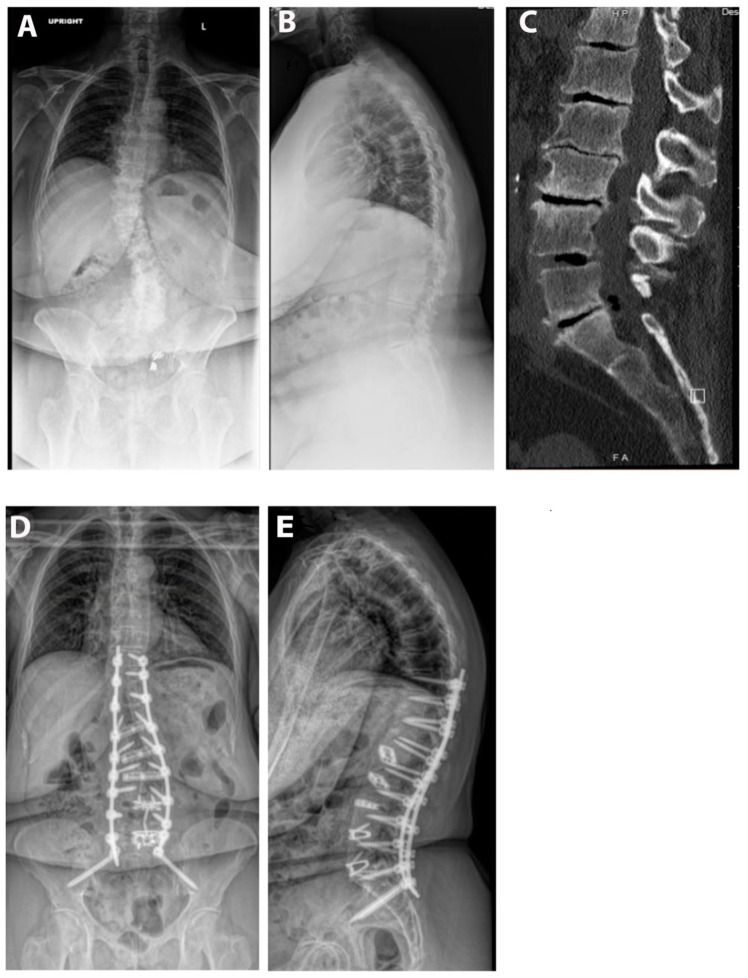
Example case. The patient is a 67-year-old male with a BMI of 36. He was having severe back and leg pain and had attempted extensive conservative measures. His pre-op SVA was 11 and PI-LL mismatch was 35°. He underwent an L4-S1 ALIF and then a L2-5 prone lateral with T10-pelvis posterior fusion. (A) Pre-op upright AP X-ray. (B) Pre-operative upright lateral X-ray. (C) Pre-operative CT scan. (D) AP postoperative upright AP X-ray. (E) Postoperative upright lateral X-ray.
